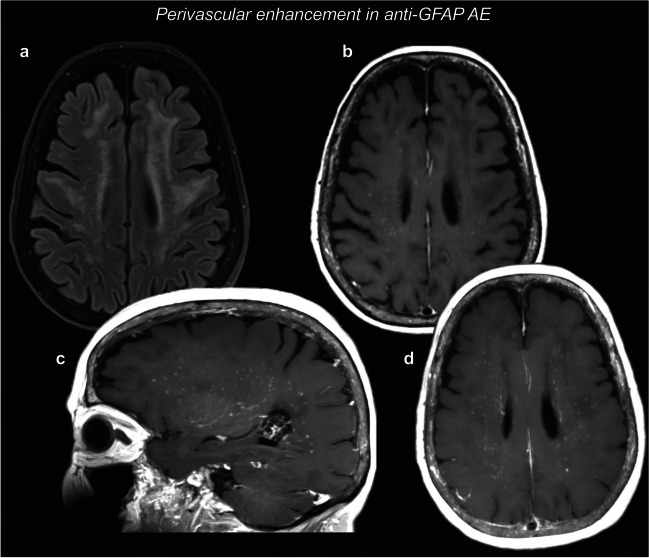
Perivascular enhancement in the centrum semiovale. T2-weighted FLAIR images (a) showed widespread signal alterations in the bi-hemispheric white matter. Post-contrast T1-weighted images (b) showed numerous spots of perivascular enhancement in the centrum semiovale, which can be more easily appreciated with maximum intensity projection (MIPs) images (c, d). Anti-GFAP autoantibodies were positive in CSF and serum
